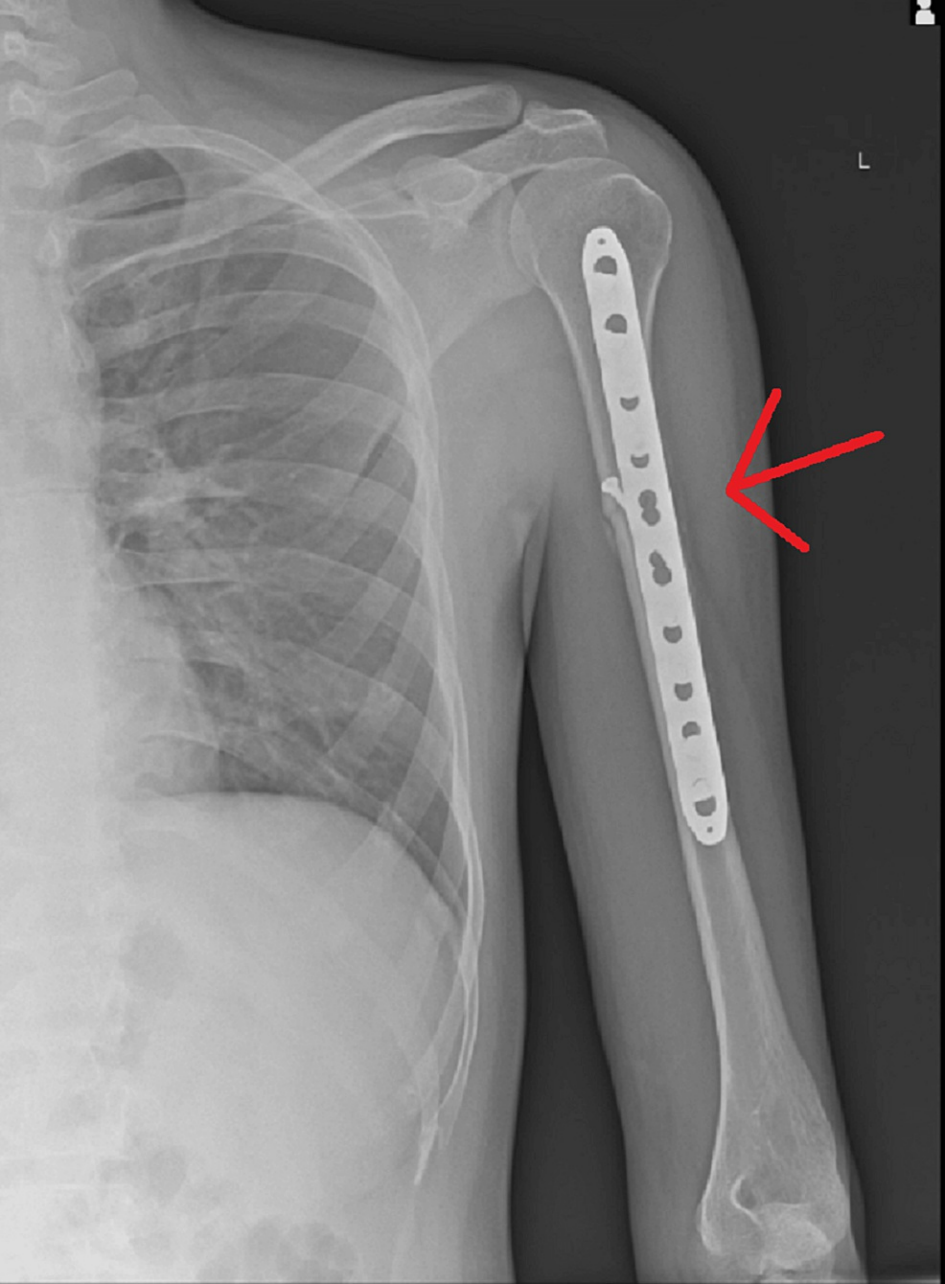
Post-one-month radiograph showing ORIF with plate osteosynthesis for proximal one-third humerus shaft fracture on left side ORIF - open reduction and internal fixation
